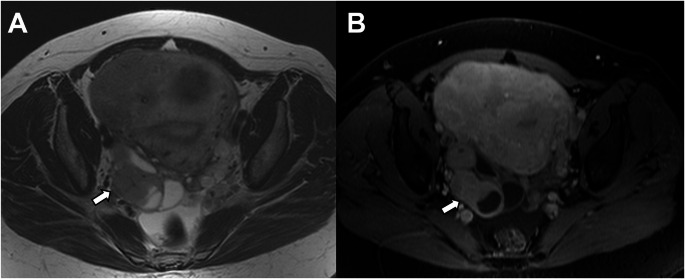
Stage IIIA1 carcinosarcoma in a 72-year-old woman. A T2-weighted fast spin-echo (FSE) image (TR/TE: 4500/100 ms; slice thickness: 3 mm) and B axial contrast-enhanced 3D T1-weighted fat-suppressed LAVA sequence (TR/TE: 4.2/2.1 ms; slice thickness: 2 mm; post-gadolinium, delayed phase at 4 min) show absent myometrial signal intensity and a right adnexal mass (arrow). Pathology confirmed involvement of the myometrium and right adnexa by carcinosarcoma
